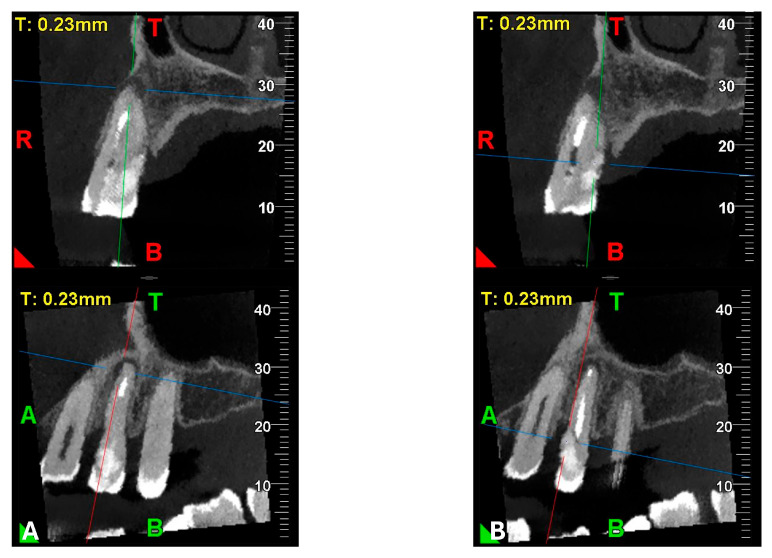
Pano-rex and cross-sectional CBCT images of tooth 1.4 after six months of healing. (A,B) Sequential scrolling of tooth 1.4 after six months of healing. The colored lines crossing the images represent the orthogonal planes (axial, coronal, and sagittal) in the multiplanar reconstruction. Their correct use and orientation allow for an accurate identification of the extent of the lesions in all spatial planes and a detailed analysis of the endodontic anatomy. The green and red letters are the coordinates of the image: A: anterior, R: right, B: bottom, T: Top.
